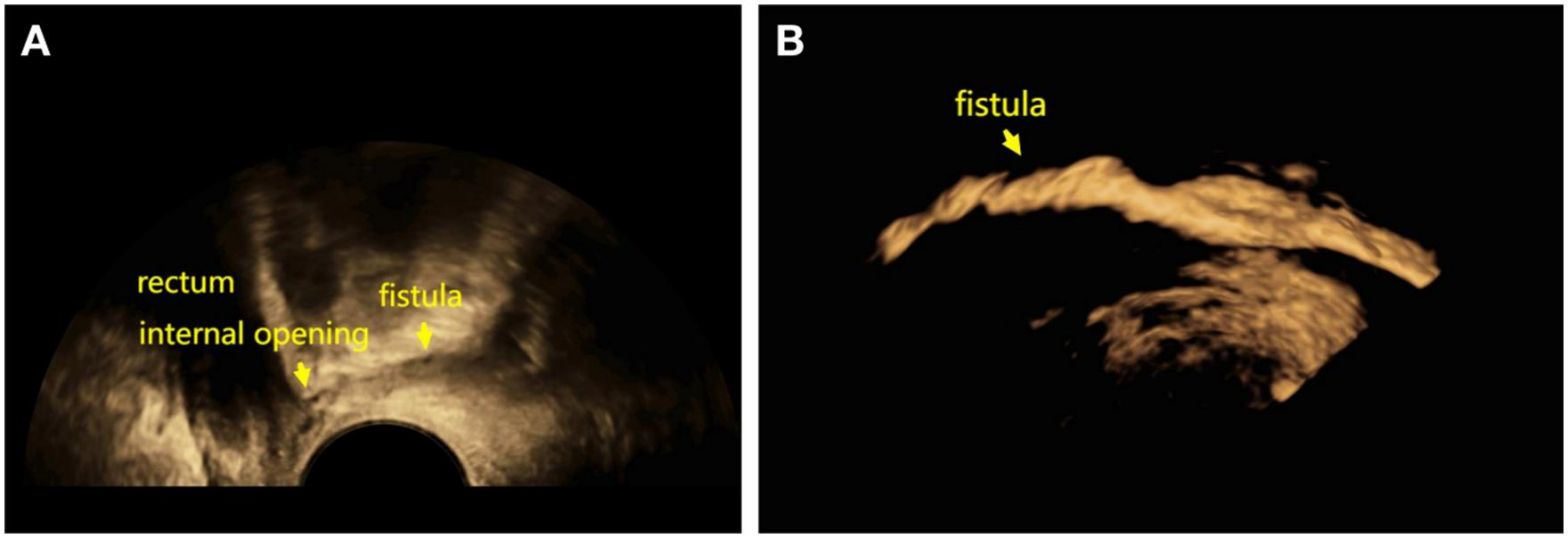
Fistula imaging under ultrasound. (A) The fistula is seen as a linear hypoechoic tract. (B) The fistulous tract turns lustrously bright and reflective after injection of SonoVue suspension.
